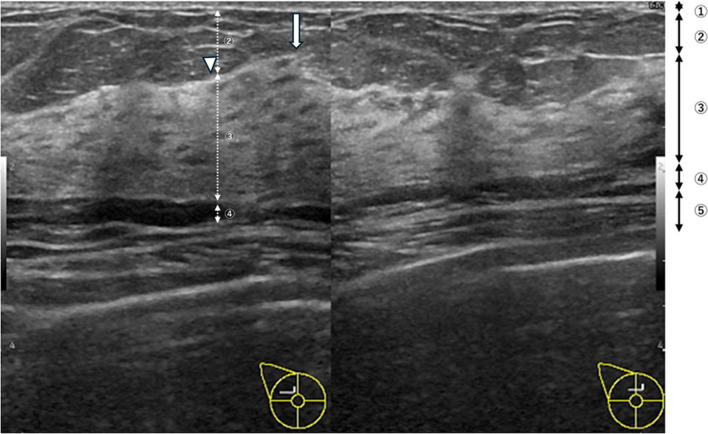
Routine breast ultrasonography examination image of the right breast. The numbers on the image correspond to the numbered anatomical structures: 1, skin; 2, subcutaneous fat; 3, fibroglandular tissue (FGT); 4, retromammary fat; and 5, chest muscle. This image does not show any site with flat anterior superficial fascia including the thickest FGT layer. The better representative point (arrowhead) is halfway between a site with a concave anterior superficial fascia and a site with a convex anterior superficial fascia due to the Cooper ligament. FGT thickness-to-FAT thickness ratio = FGT thickness (3)/FAT thickness (2 + 4). FAT means subcutaneous fat (2) and retromammary fat (4). The arrow indicates a site with a convex anterior superficial fascia due to the Cooper ligament that is not suitable for the representative point
